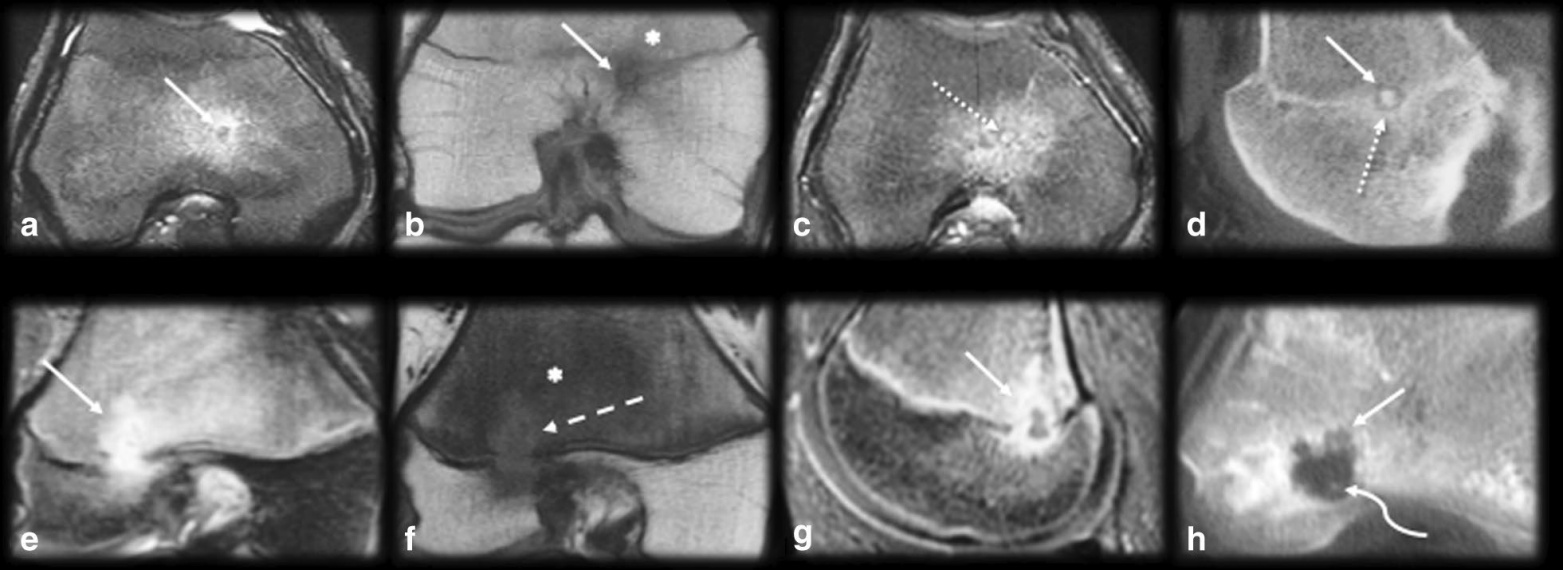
Osteoid osteoma versus osteomyelitis. (a–d) Male, 15 years old, presenting with knee pain for 2 months. MRI T2 FS (a), T1 (b), T1 FSGD (c) and CT (d) showed a nidus with smooth margins (arrows), a central mineralized portion (dashed arrows), homogeneous gadolinium enhancement (dotted arrows) and hazy T1 bone marrow edema around the lesion (asterisk). (e–h) Male, 13 years old, presenting with knee pain for 6 weeks. MRI T2 FS (e), T1 (f), T1 FS GD (g) and CT (h) showed a bone abscess with irregular margins and peripheral enhancement (arrows). Note there was mild bone marrow edema (asterisk), a positive penumbra sign (dashed arrow in f) and a small peripheral bone sequestrum (curved arrow in h)
